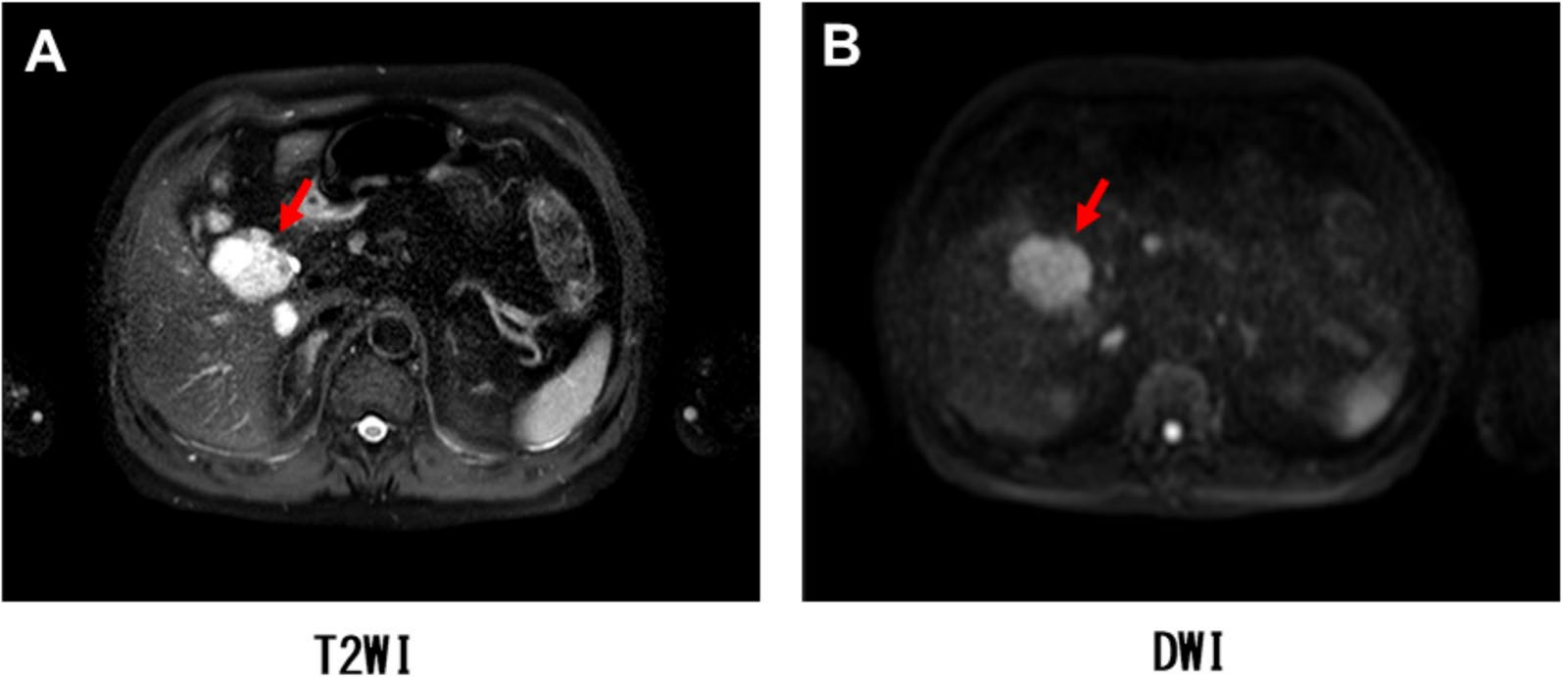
Abdominal magnetic resonance imaging. A A mass with a clear border, including hyperintense cystic and reticulated structures on T2-weighted imaging (arrow). B A mass with a hyperintense region on diffusion-weighted imaging (arrow)
